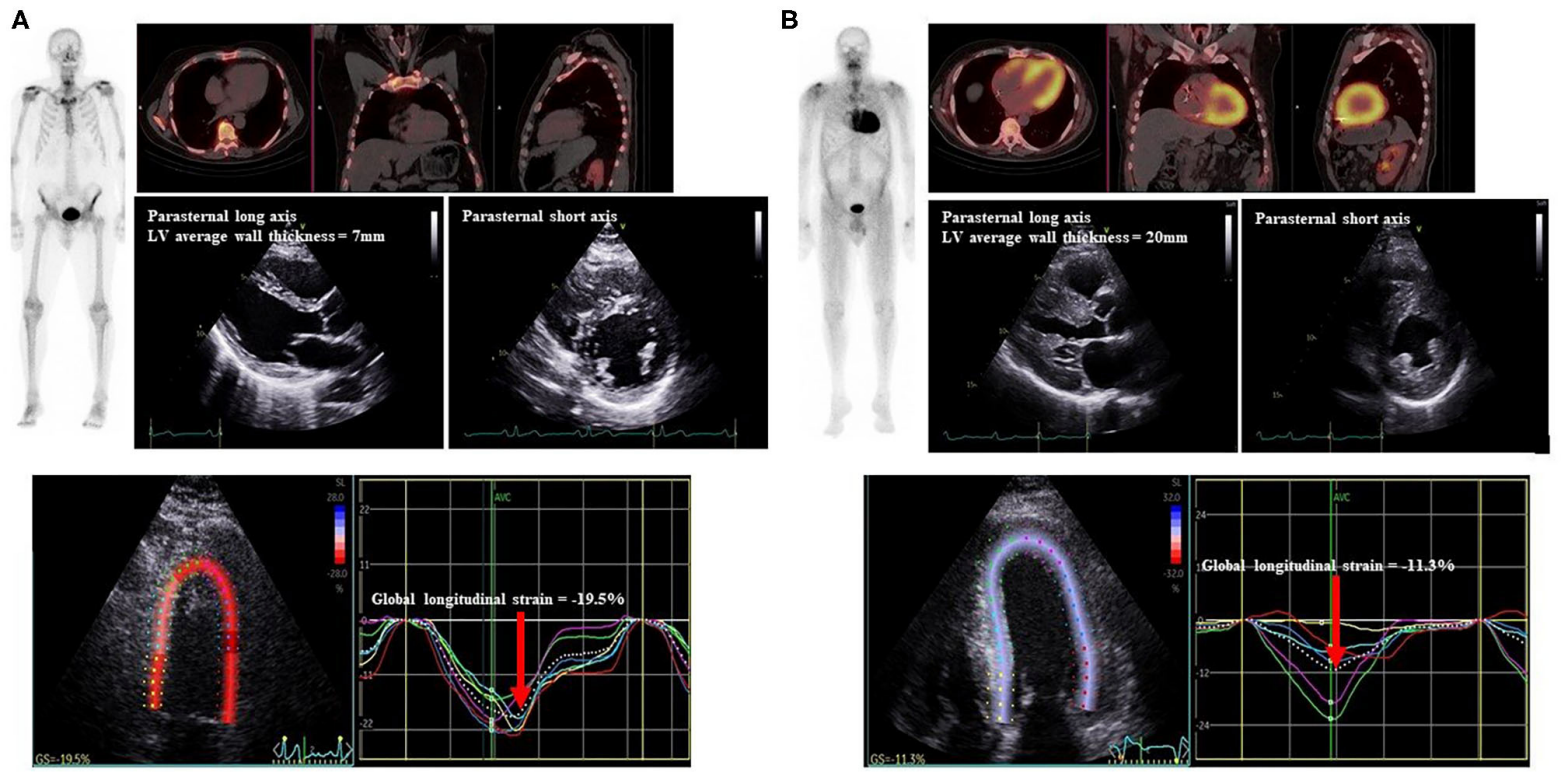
Representative Echocardiogram and ^99m^Tc-DPD scintigraphy of study patients. Echocardiograms with parasternal long and short axis views with LV wall thickness measured, demonstration of LV GLS (red arrow), ^99m^Tc-DPD whole body sweep and SPECT-CT demonstrating (A) negative scintigraphy, normal LV wall thickness and GLS and (B) positive scintigraphy, increased LV wall thickness and reduced LV GLS. ^99m^Tc-DPD, Technetium-99m 3,3-diphosphono-1,2-propanodicarboxylic acid; LV, Left ventricle; GLS, global longitudinal strain; SPECT-CT, single-photon emission computed tomography-computerized tomography.
